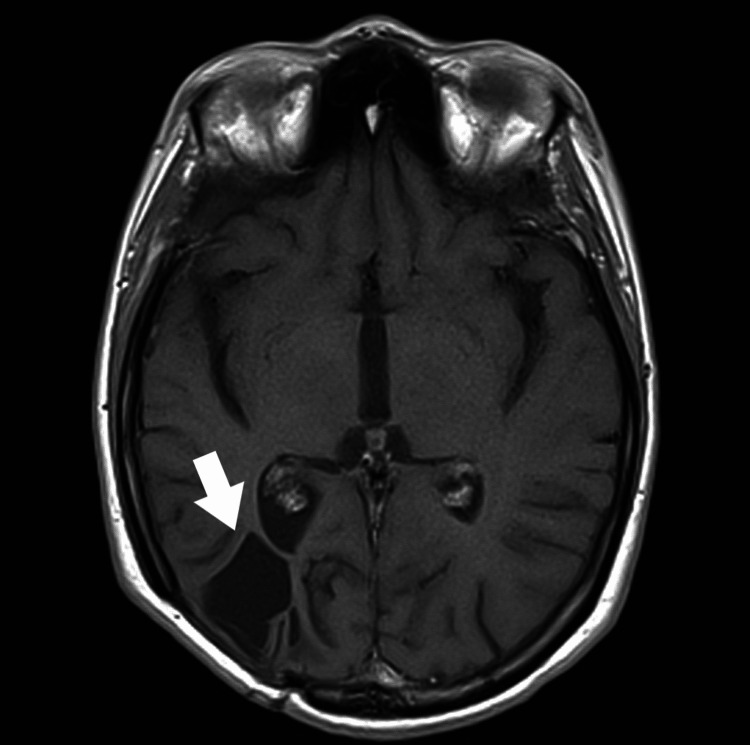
A follow-up MRI scan of the head three years after surgery: a top view. No signs of recurrence of the abscess (marked with a white arrow).
